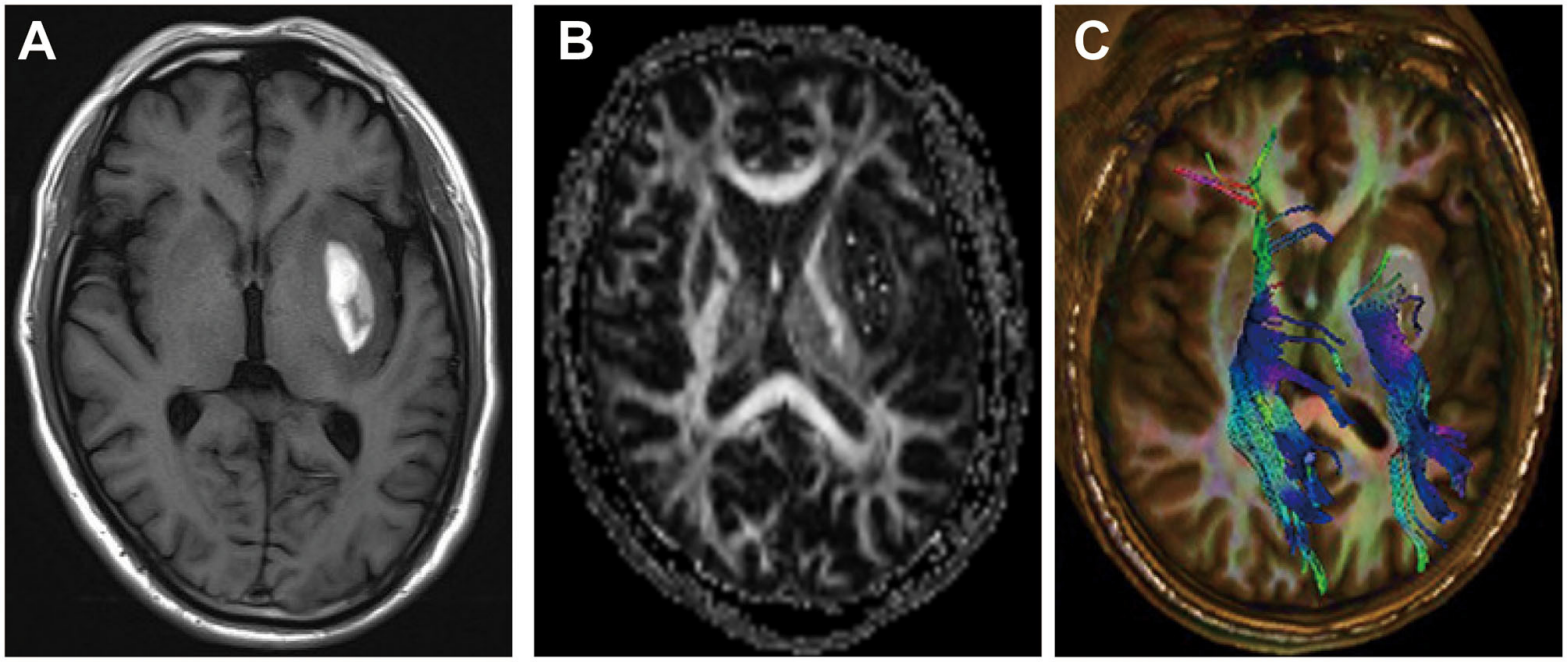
Example of white matter injury (WMI) after intracerebral hemorrhage (ICH). (A) T1-weighted image after ICH. (B,C) Diffusion tensor images of WMI after ICH.
